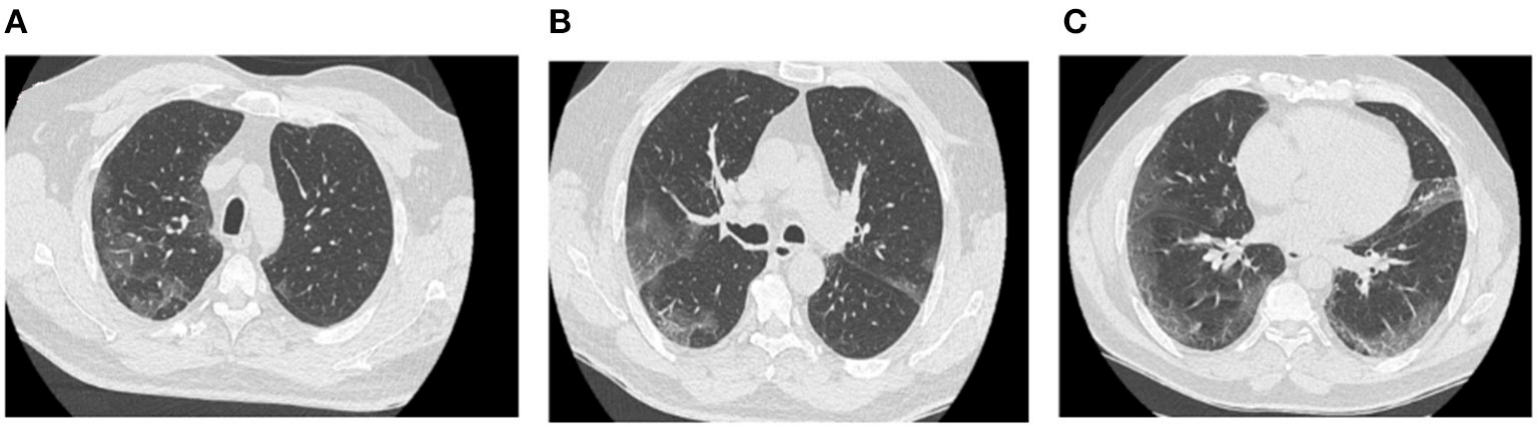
Chest CT scan of COVID-19 pneumonia (first chest CT scan). Axial sections of the chest CT scan at different levels show bilateral ground-glass opacities and consolidation images that are bilateral, subpleural, and located mainly in posterior regions (A–C).
